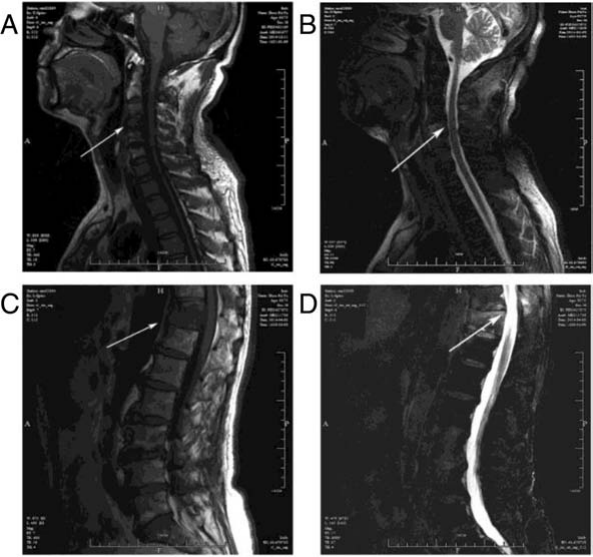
MRI image of the patient with multifocal musculoskeletal tuberculosis. a MRI showed degeneration in cervical vertebrae. T1-weighted sagittal image showed heterogeneous, mostly hypointense, lesions involving the C4 and C5 vertebrae (*arrowhead*). b T2-weighted sagittal image showed posterior protrusion of C3-4, C4-5, C5-6 and C6-7 and light compression of the corresponding meninges capsule (*arrowhead*). c MRI showed degeneration of thoracic vertebra. T1-weighted sagittal image showed heterogeneous, mostly hypointense, lesions involving T11 (*arrowhead*). d T2-weighted sagittal image showed posterior protrusion of T11 and light compression of the corresponding meninges (*arrowhead*)
